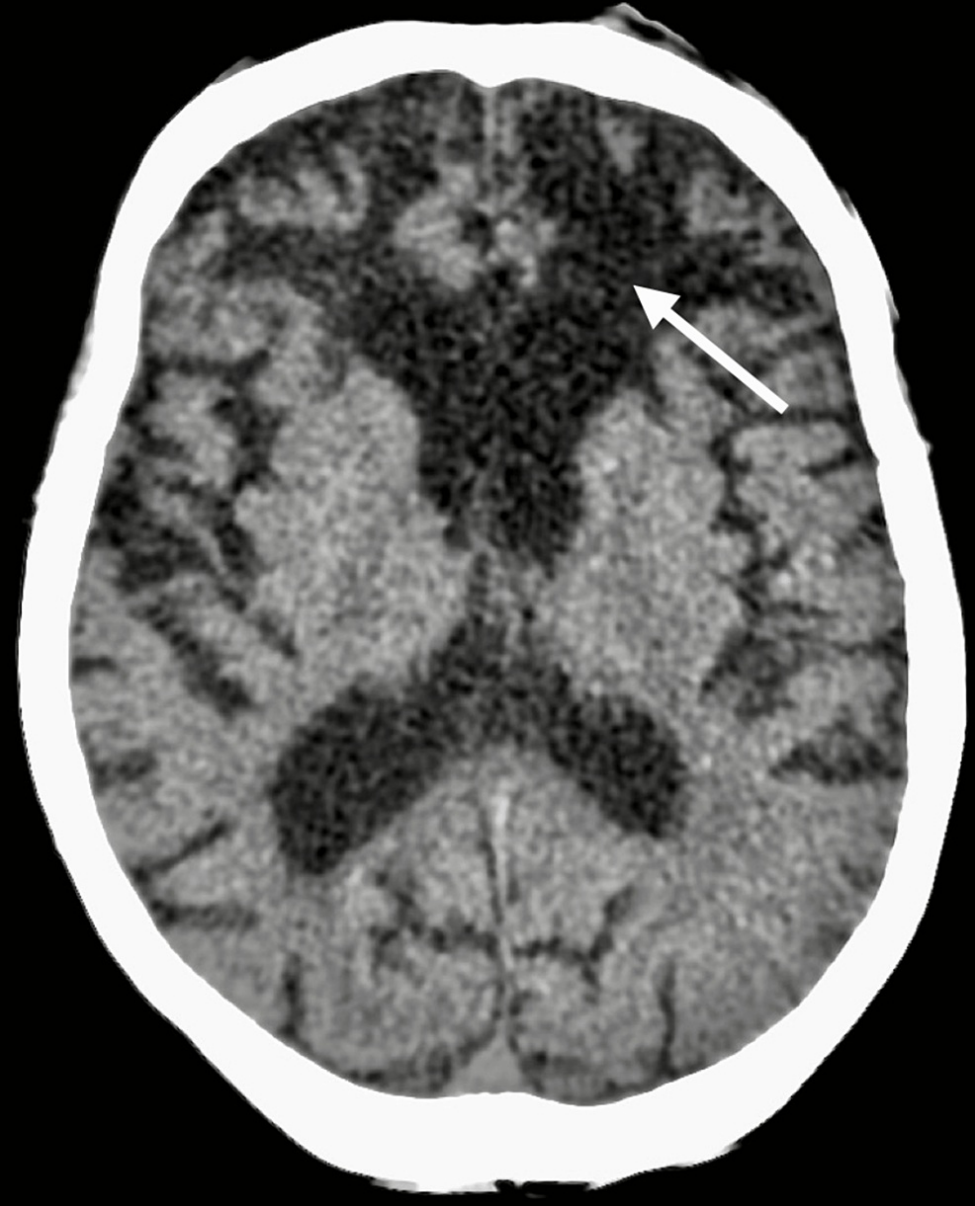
CT scan showing old contusion trauma sequels and a pattern of cortical-subcortical atrophy
